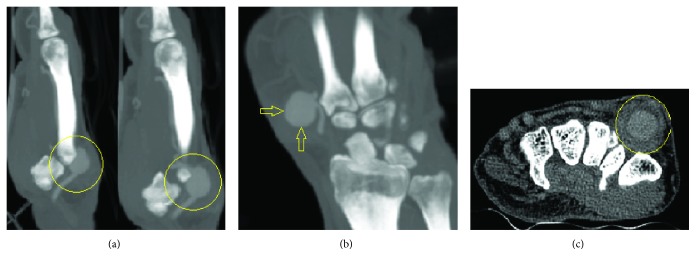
Computed Tomography Angiography shows the 15 × 9 × 11 mm distal radial artery aneurysm (yellow circles and arrows) in sagittal (a), coronal (b), and transverse (c) sections.
